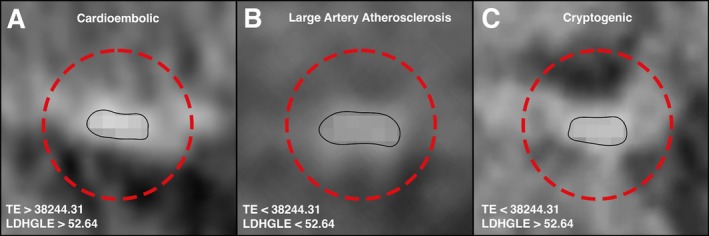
NCCT imaging analysis. Radiomics‐based analysis of clots from various stroke etiologies revealed distinct compositions. (A) Clots with TE and LDHGLE values exceeding the 70th percentile were predominantly rich in RBCs, a characteristic pattern observed in cardioembolic strokes. (B) In contrast, clots associated with large artery atherosclerosis exhibited TE and LDHGLE values below the established RBC‐rich threshold, indicating a lower RBC content. (C) Interestingly, cryptogenic clots demonstrated elevated LDHGLE values above the RBC threshold, suggesting a composition similar to cardioembolic clots, with a high proportion of RBCs. LDHGLE = large dependence high gray level emphasis; NCCT = non‐contrast computed tomography; RBCs = red blood cells; TE = total energy. [Color figure can be viewed at www.annalsofneurology.org]
